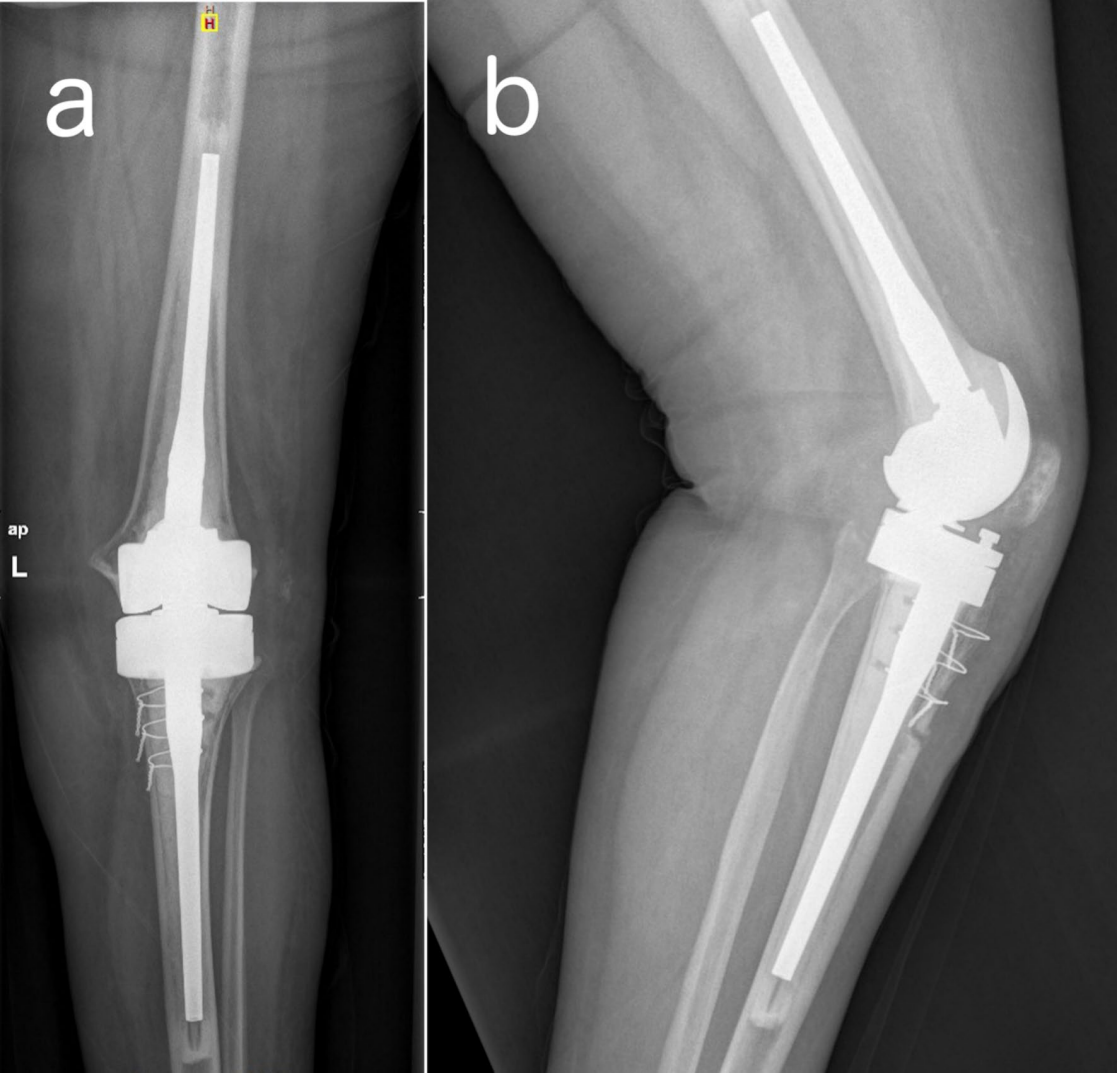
a Anteroposterior and b lateral post-operative radiography showing an Endo-Model®-M knee revision prosthesis
